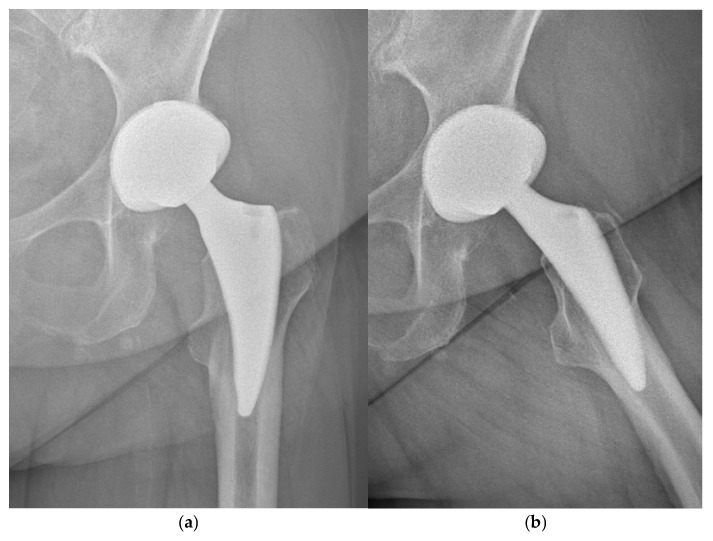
(a,b): Anteroposterior and axial radiographs of the same patient, 6 years postoperatively, show osseously stable stem fixation with visible femoral cortical hypertrophy in Gruen zones 3–5 (around the distal part of the stem) and a grade I stress-shielding phenomenon in Gruen zone 7.
